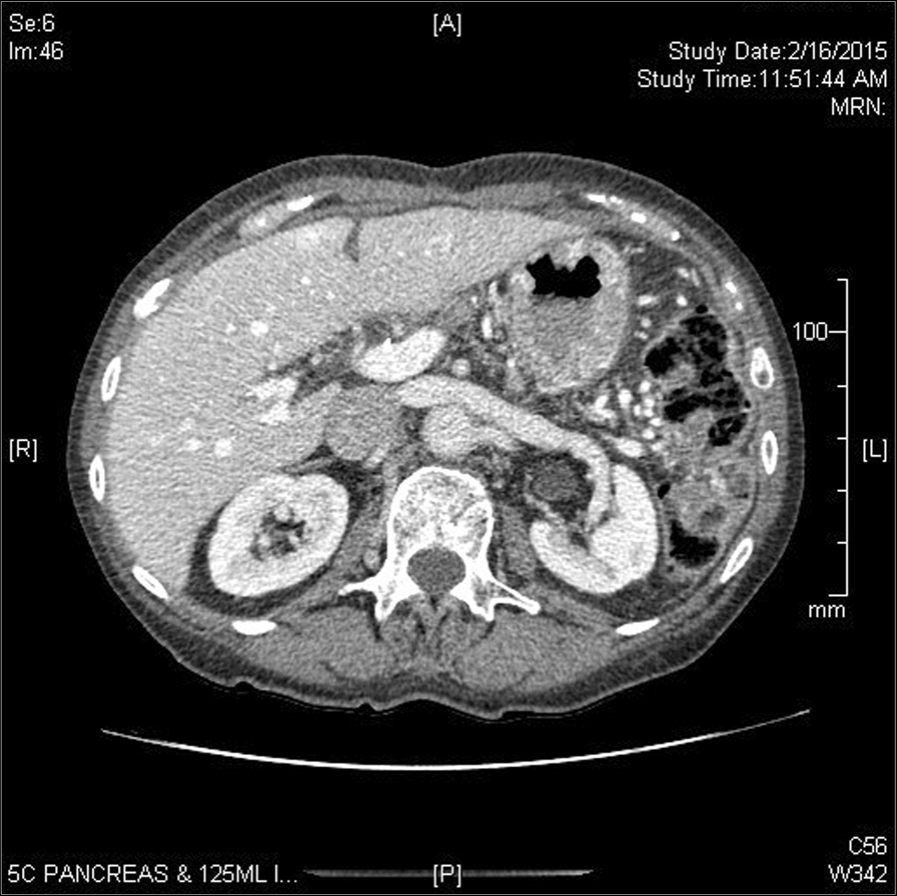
Follow-up CT 11 months after completion pancreatectomy. At this point, the patient has completed adjuvant chemotherapy and chemoradiation and there is no evidence of disease
